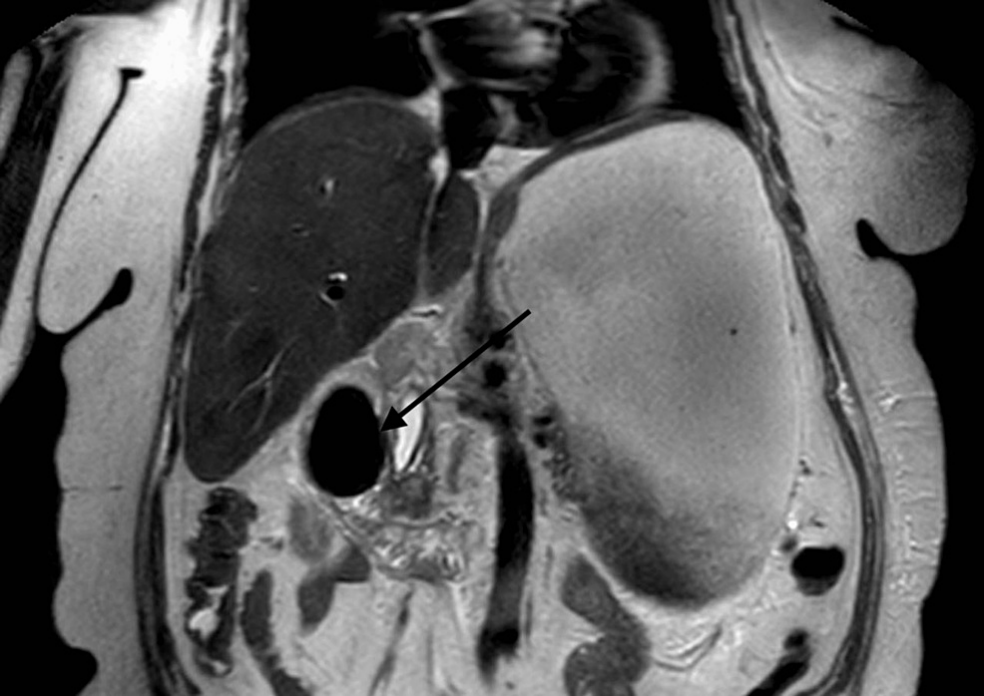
MRI showing giant stone in second part of duodenum (black arrow) and massively distended stomach
